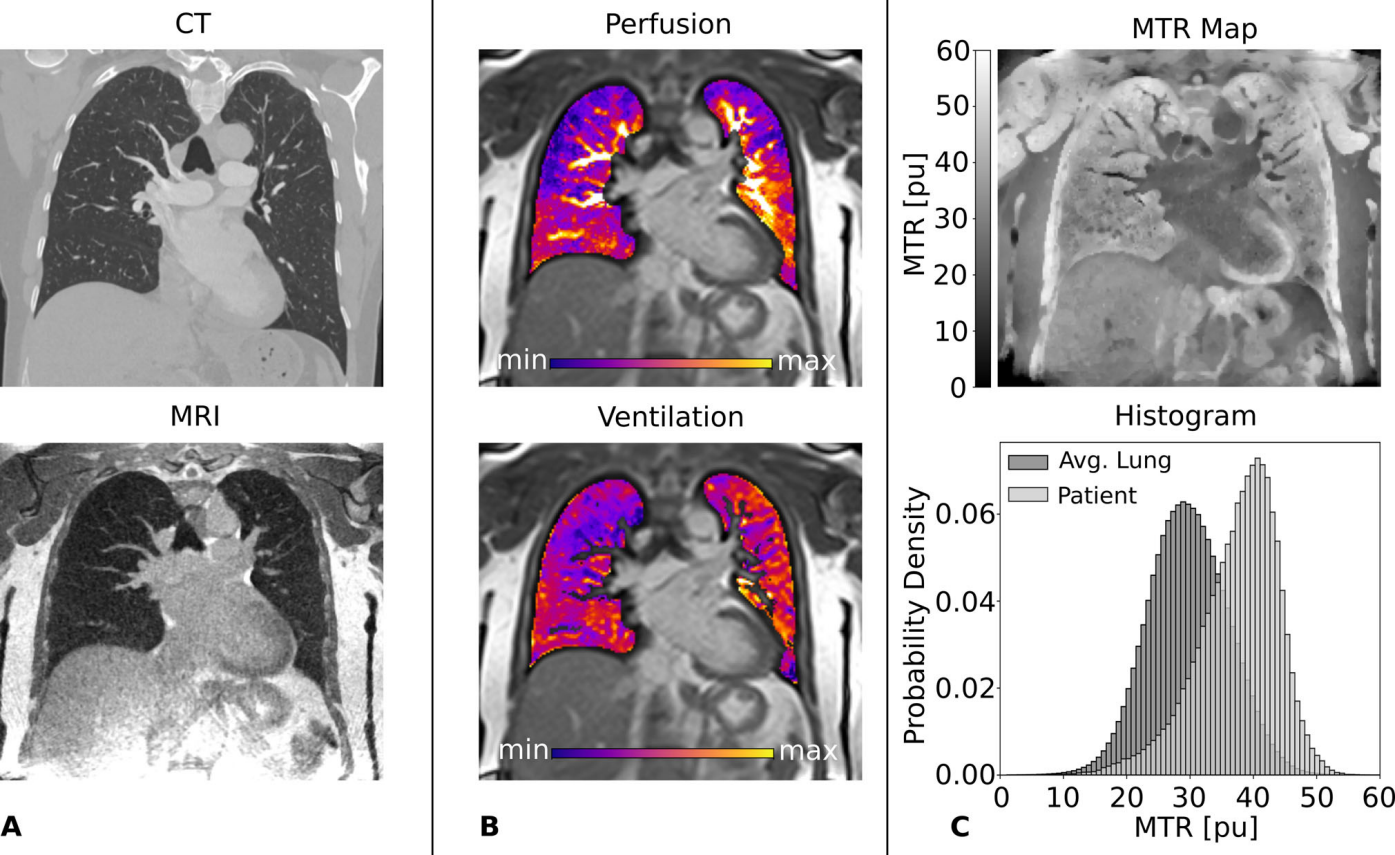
Patient with T2‐low asthma: (A) Morphological images: CT and MRI, (B) functional maps: perfusion‐weighted MP MRI map and ventilation‐weighted MP MRI map, and (C) MTR maps and whole lung MTR histogram for the patient in light gray and collective data of healthy volunteers, representing an average healthy lung, in dark gray.
